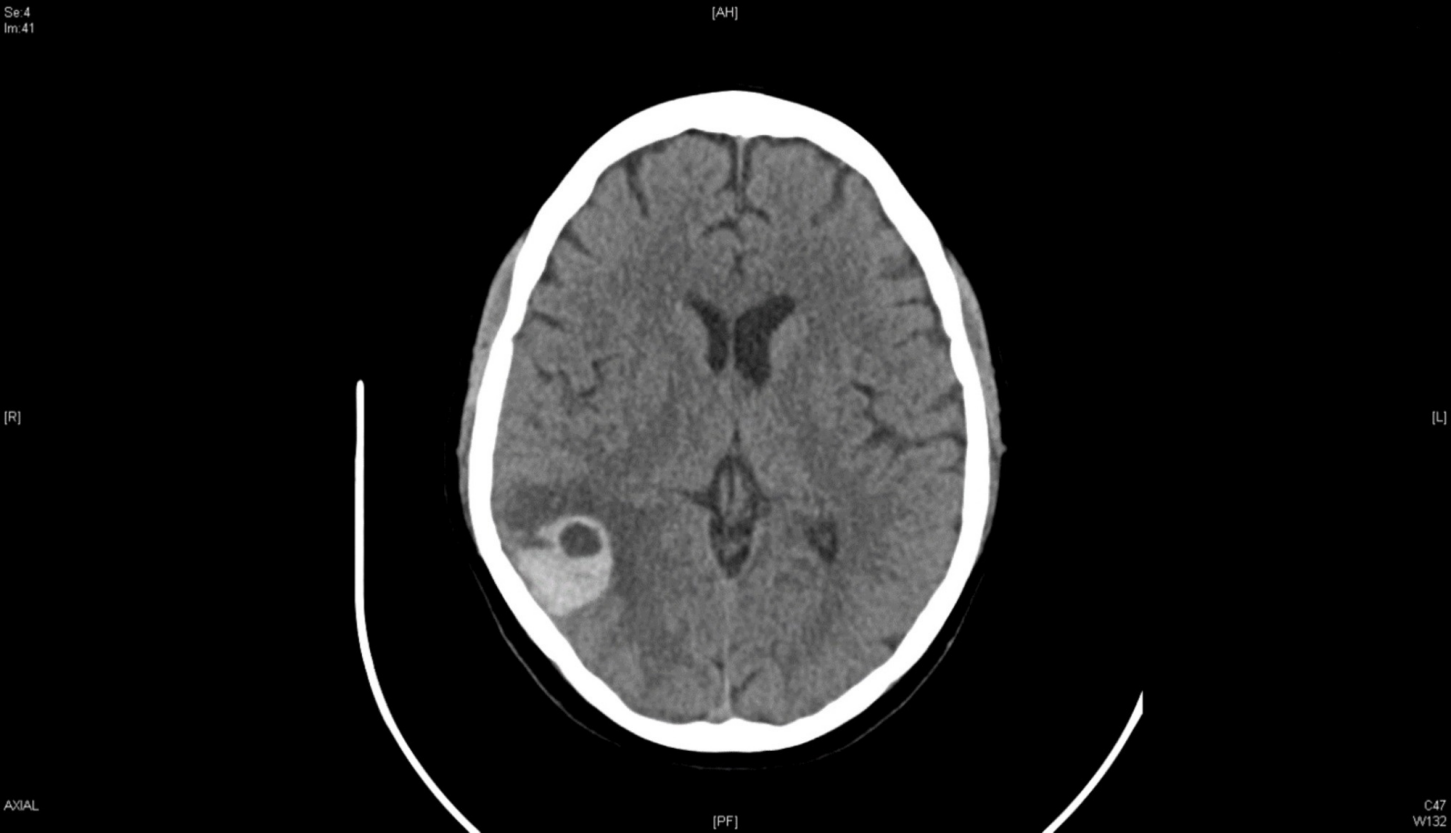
Computed tomography (CT) head without contrast, day 6.
